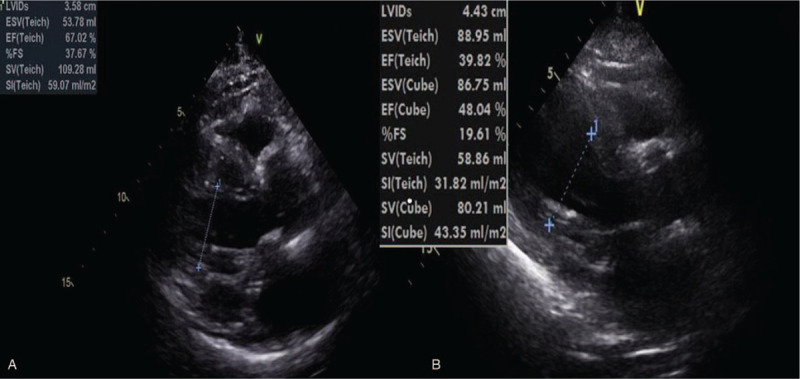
The preoperative transthoracic echocardiograph (A) showing ischemic heart disease with preserved left ventricular ejection fraction (67%) while the postoperative transthoracic echocardiograph (B) shows ischemic heart disease with moderate left ventricular systolic dysfunction (ejection fraction = 39%). EF = ejection fracture, ESV = endsystolic volume, FS = fractional shortening, LVID = Left ventricle internal diameter, SI = stroke index, SV = stroke volume.
